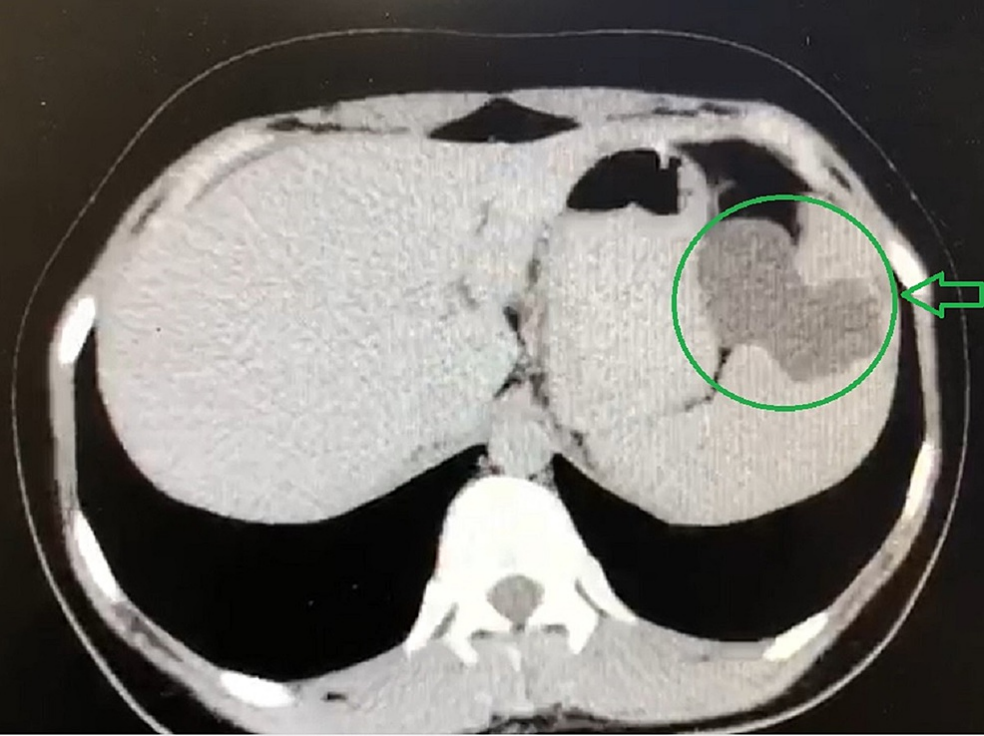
Axial view of the splenic hydatid cyst on abdominal CT scan The green arrow shows the location of the splenic hydatid cyst
